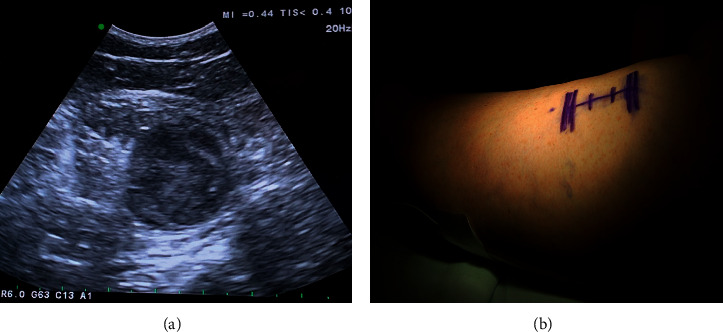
(a) Intraoperative ultrasound localizing tumor. (b) Planned incision along the right posterior thigh.
